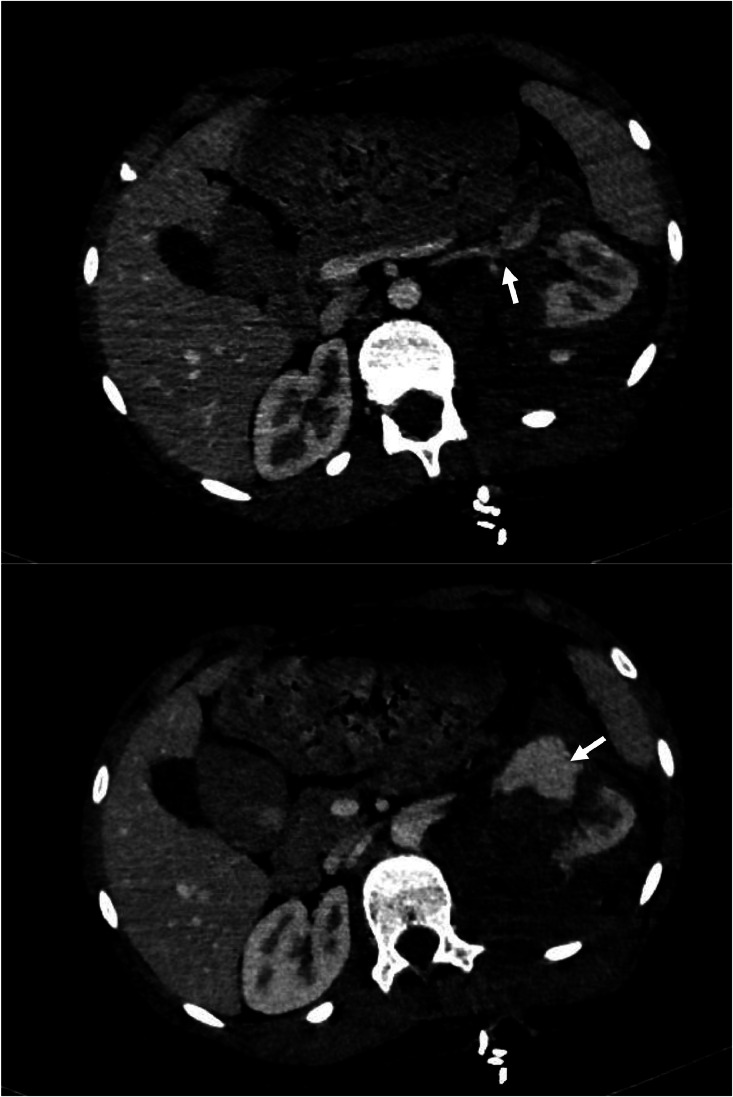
Axial contrast-enhanced abdominal CT showing rupture of the left renal vein (A) with active contrast extravasation (B).
